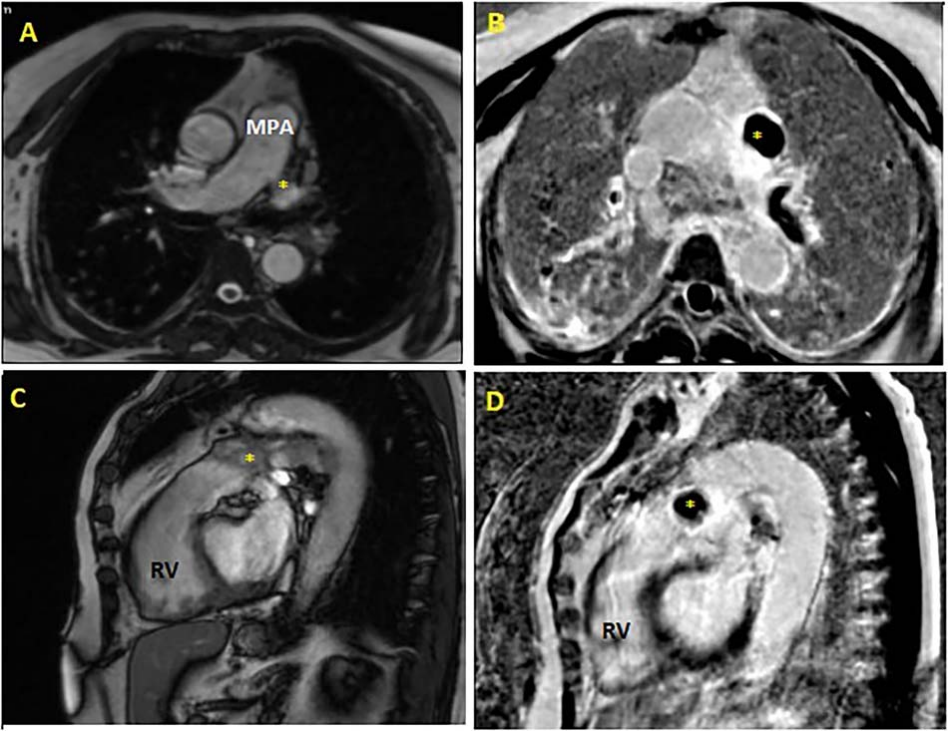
Cardiac MRI: cine axial view (A) and cine sagittal view (C) revealing intraluminal active multivesicular honeycomb-like cyst in the left pulmonary artery, hypo-intense in LGE sequences (axial view: B, sagittal view: D). MPA: main pulmonary artery; RV: right ventricle.
